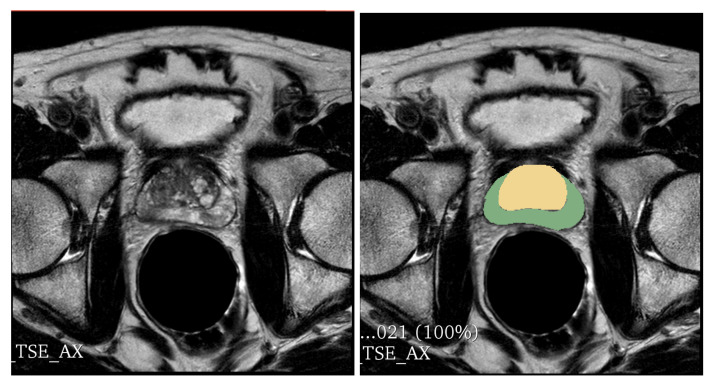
MRI slices related to a patient affected by 4 + 3 Gleason score prostate cancer.
